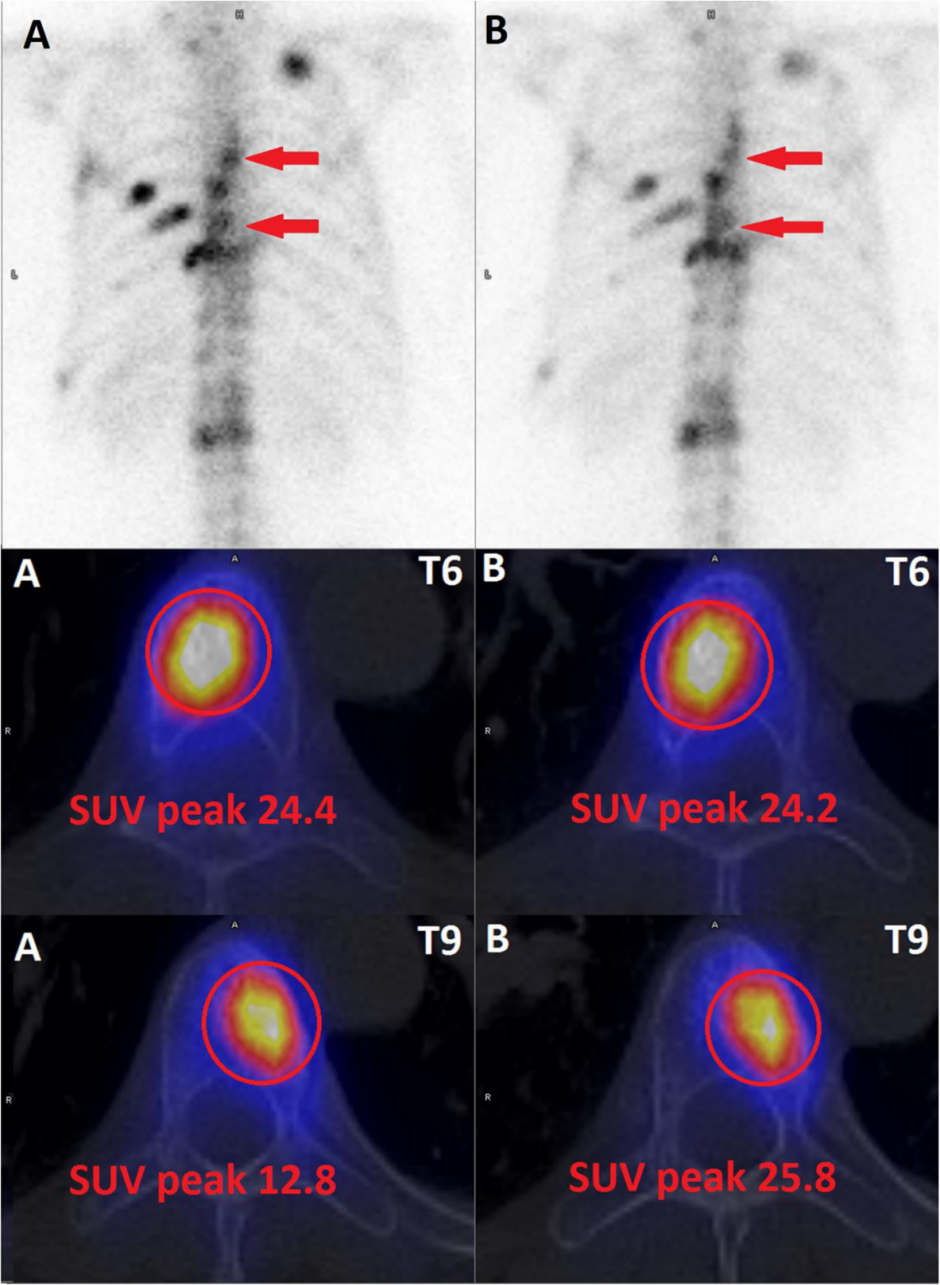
Planar bone scintigraphy and fused SPECT/CT at TP1 (a) and TP2 (b) of a patient with metastatic breast cancer. Both metastases in the thoracic spine were visually considered stable. Quantitative assessment revealed an increased tracer uptake for the lesion in thoracic vertebra body 9 (T9), whereas activity concentration of the lesion in thoracic vertebra body 6 (T6) was stable
